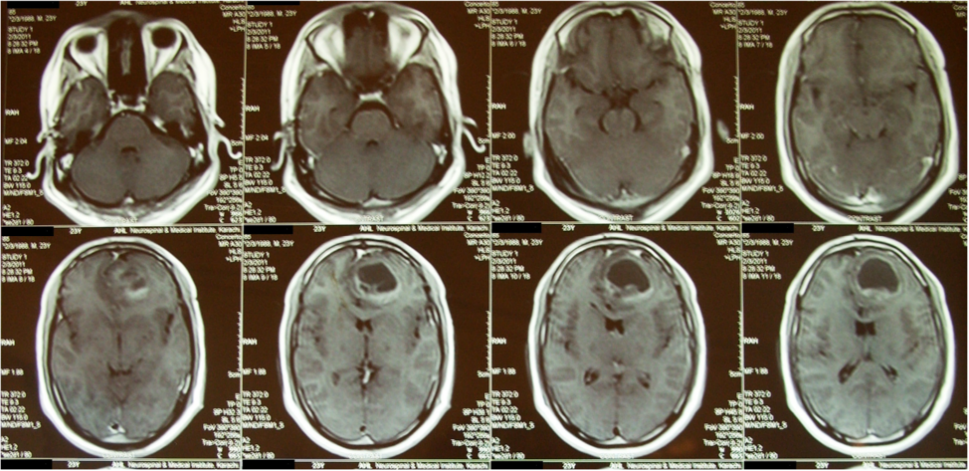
Non-contrast T1-weighted axial magnetic resonance images revealing chondroma in the left frontal lobe. Magnetic resonance images of the head conducted 3 days before surgery.
